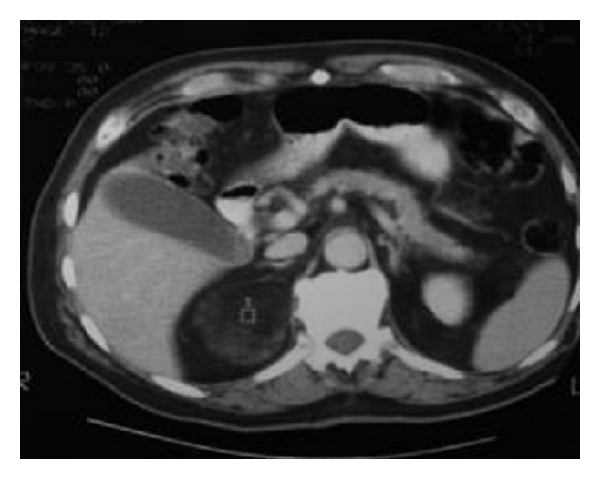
CT of the abdomen with contrast demonstrating large low-attenuation mass right adrenal gland.
